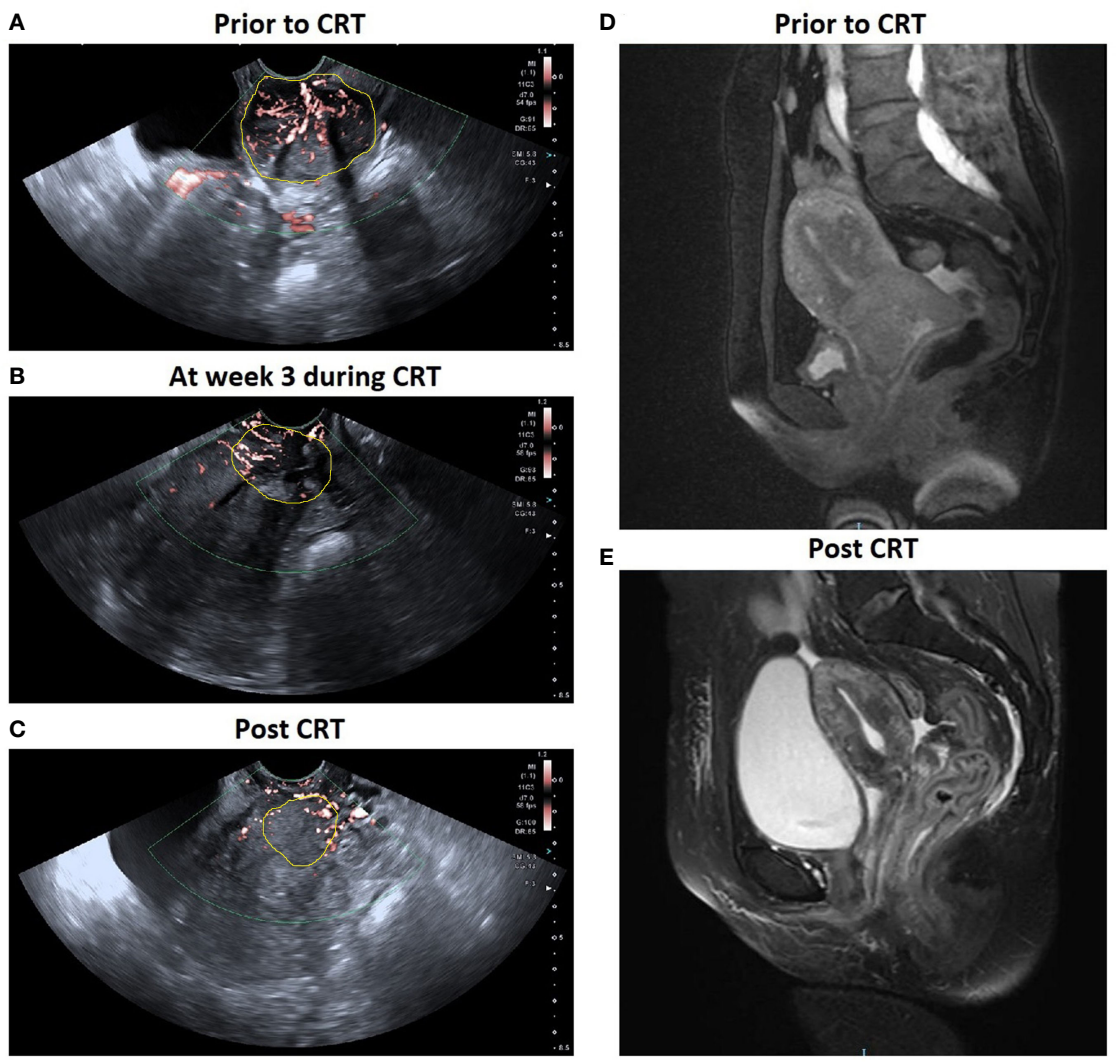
A patient with locally advanced cervical cancer (FIGO stage IIIB) experienced complete response to chemo-radiotherapy (CRT). SMI images show a significant decrease in VI in cervical cancer: (A) 0.367 prior to CRT; (B) 0.188 at week 3 during CRT; (C) 0.600 post CRT. Corresponding axial T2-weighted images exhibited a significant decrease in the maximal diameter of tumor: (D) 5.3 cm at pre-therapy e and (E) 0 cm post therapy.
